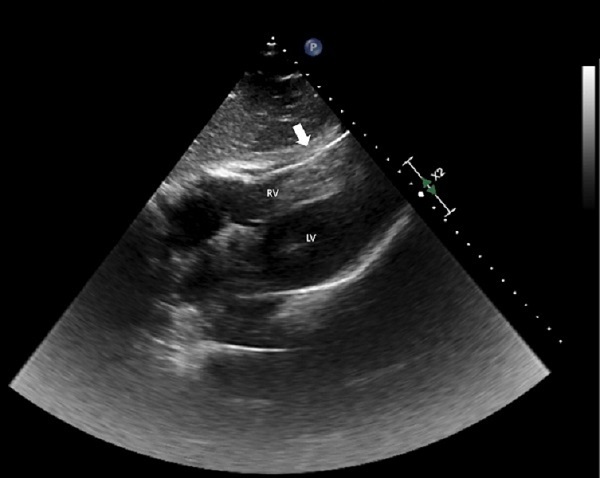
Point-of-care echocardiogram subcostal view during systole showing ventricular pacer lead (arrow) beyond right ventricular chamber. No pericardial effusion is seen. RV, right ventricle; LV, left ventricle.
